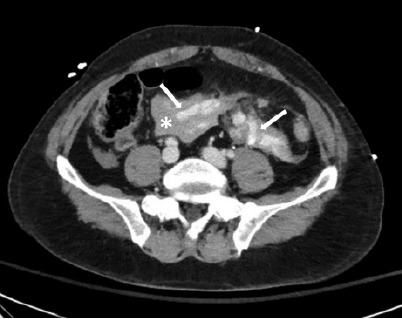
Abdominal computed tomography (axial image) with active extravasation of contrast (arrows) and free fluid (asterisk) in the lower abdomen suggestive of mesenteric injury.
